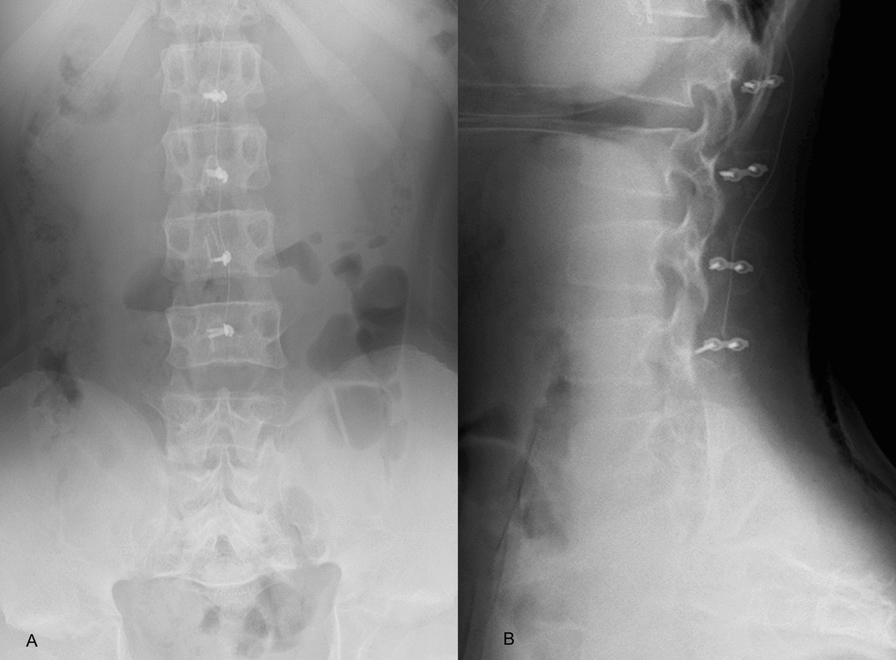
Postoperative lumber X-ray images (A: lateral view, B: frontal view). Spinous process reduction laminotomy (T12–S1) was performed. The detached spinous processes, except the L5 spinous process, were reattached via mini plate–screw fixation. The L5 spinous process was secured with surgical sutures because the plate was difficult to place because of hyperlordosis
